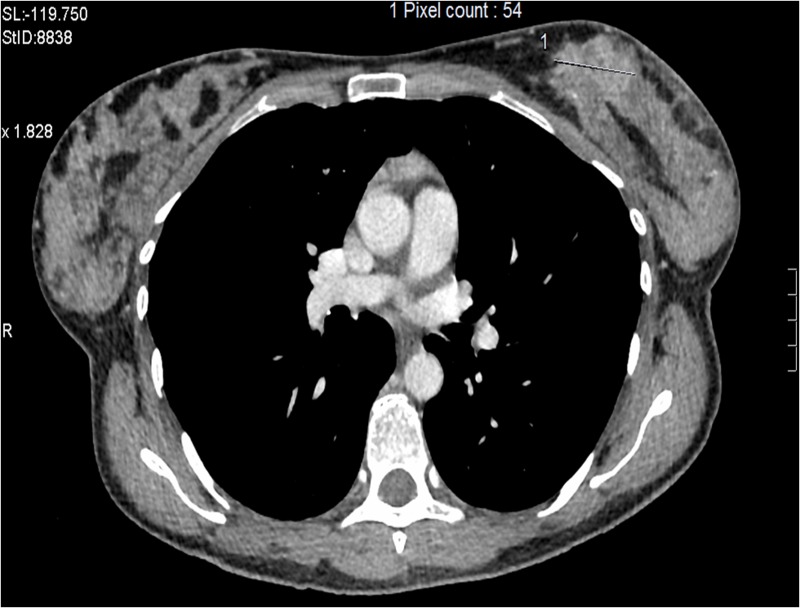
Patient 1: Baseline computed tomography (CT) at diagnosis reveals left breast mass
